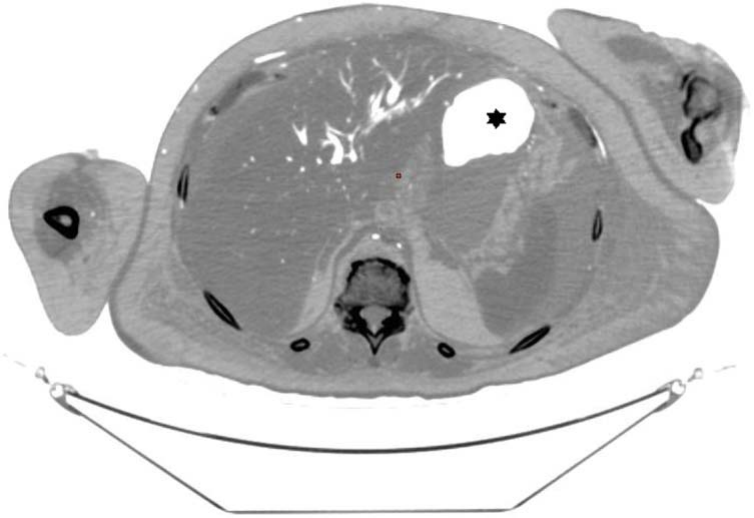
Axial CT (inverse grey scale): The hepatic parenchyma shows mostly intravascular air filling the intrahepatic vasculature and possibly minute dots of parenchymal air. The area marked with a star represents normal gastric air.
